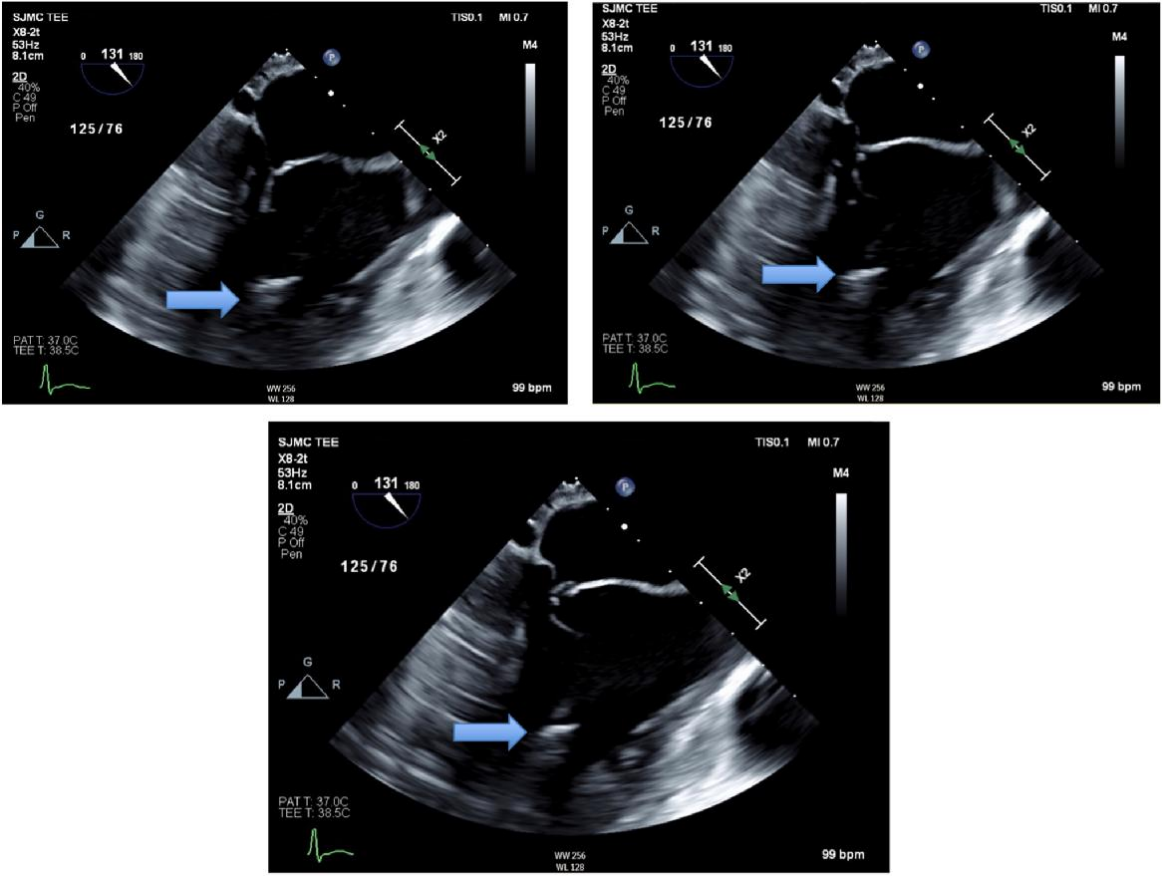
Transesophageal echocardiogram showing 1.5-cm filamentous mobile mass on the ventricular side of the mitral valve leaflet, suggestive of vegetation.
